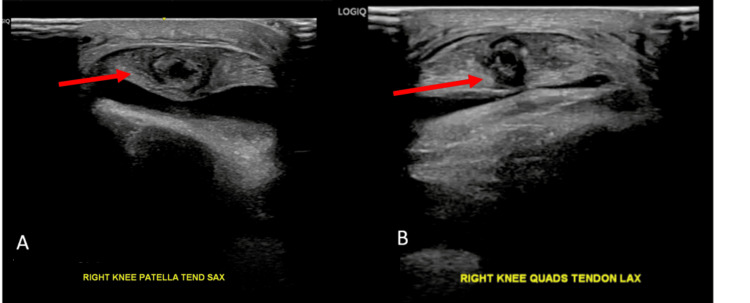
Ultrasound images of the right knee showing (A) hypoechogenic changes within the substance of the PT in keeping with an intrasubstance tear and (B) a hypoechogenic compressible substance seen on the ultrasound, which was eventually aspirated and had a chalky appearance
